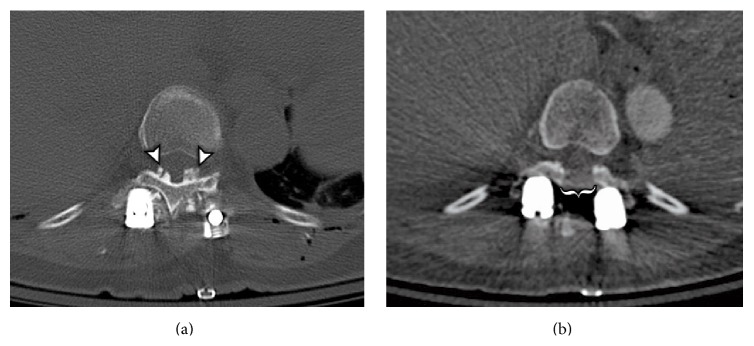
Postfacetectomy (a) and postlaminectomy (b) axial Computed Tomograms at the level of T10-11. The arrowheads in (a) demonstrate bilateral ossifications of the ligamentum flavum occupying the posterior aspect of the spinal canal. The bracket in (b) indicates the area where decompression was achieved via laminectomy and resection of these lesions.
